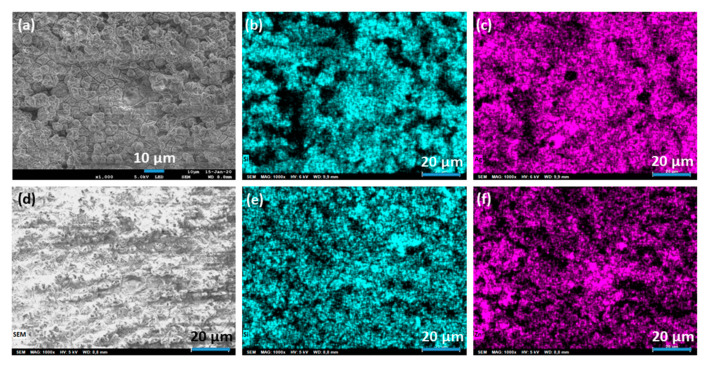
SEM images of FAU-zeolite membranes exchanged with silver (a) or zinc (d) and Energy Dispersive X-ray (EDX) mapping of silicon (b,e), silver (c) and zinc (f) elements present in these FAU-zeolite membranes.
